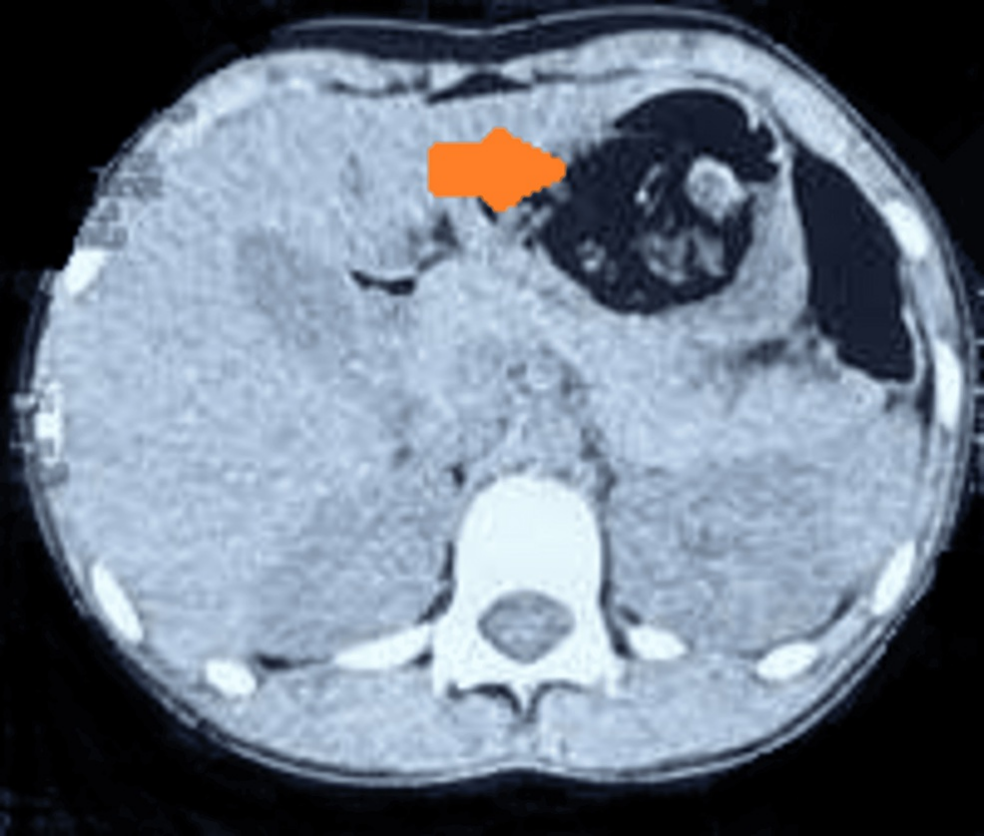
CECT abdomen showing a heterogenous irregular mass in the stomach. CECT: contrast-enhanced computed tomography
